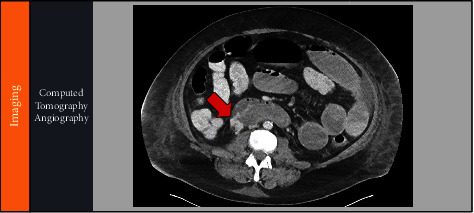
Computed tomography angiography showing a large duodenal varix with active hemorrhage into the second portion of the duodenum (red arrow).
